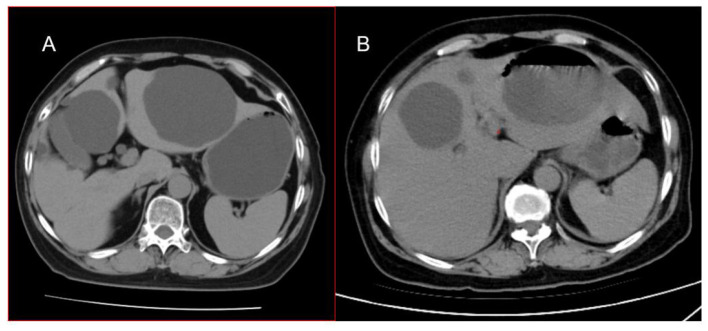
CT Imaging of Preoperative and Postoperative Sepsis in Patients with Liver Cysts, (A) Before a liver cyst aspiration, (B) After sclerotherapy for a liver cyst.
